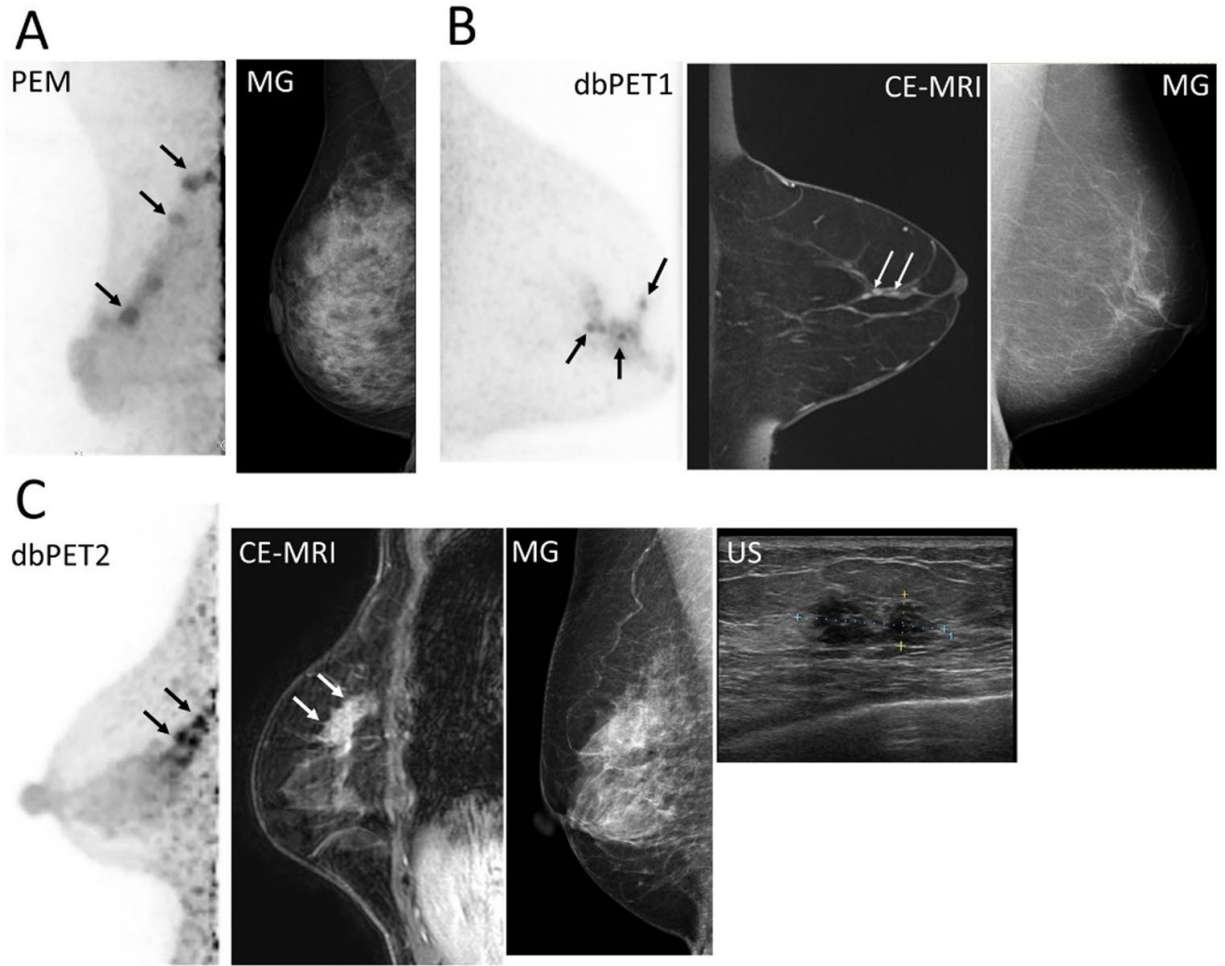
Representative breast-specific PET images for a non-mass uptake and modality differences. The primary breast cancer lesions are indicated by arrows. All PET images are displayed using an SUV scale ranging from 0 to 5. (a). A 42-year-old woman with invasive ductal carcinoma (IDC) and ductal carcinoma in situ (DCIS), luminal A). PEM and mammography (MLO). PEM was performed instead of MRI due to claustrophobia. (b). A 71-year-old woman with DCIS (intermediate type). dbPET1, contrast-enhanced MRI (delayed sagittal), and mammography (MLO). (c) A 50-year-old woman with triple-negative IDC. dbPET2, contrast-enhanced MRI, mammography (MLO), and ultrasonography. Abbreviations: PET, positron emission tomography; PEM, positron emission mammography; MG, mammography; MLO, mediolateral oblique; US, ultrasonography; IDC, invasive ductal carcinoma; DCIS, ductal carcinoma in situ; dbPET, dedicated breast PET; CE, contrast-enhanced
